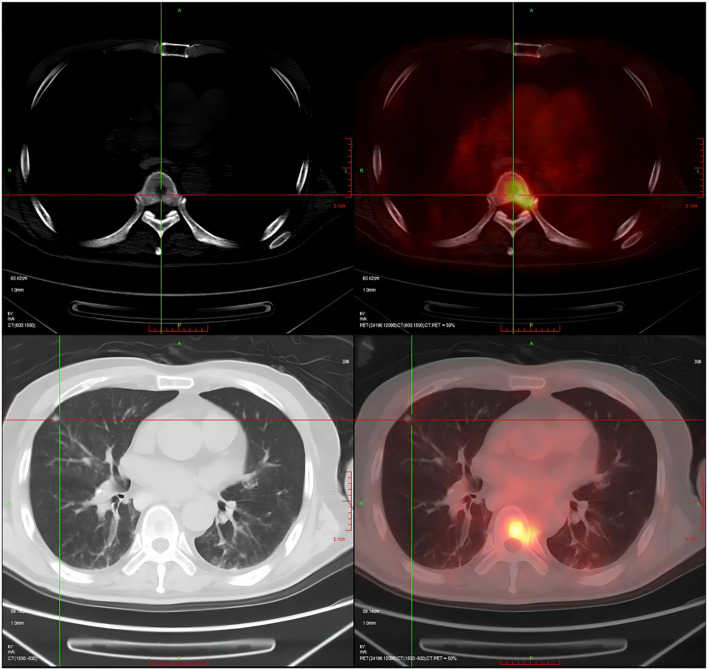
PET/CT (Positron emission tomography computed tomography) imaging revealed multiple nodules and streaks in both lungs and destructive osteolytic changes between T6 and T7, implying infectious lesions. Large bullae and calcification nodules were found in the upper lobe of the left lung
